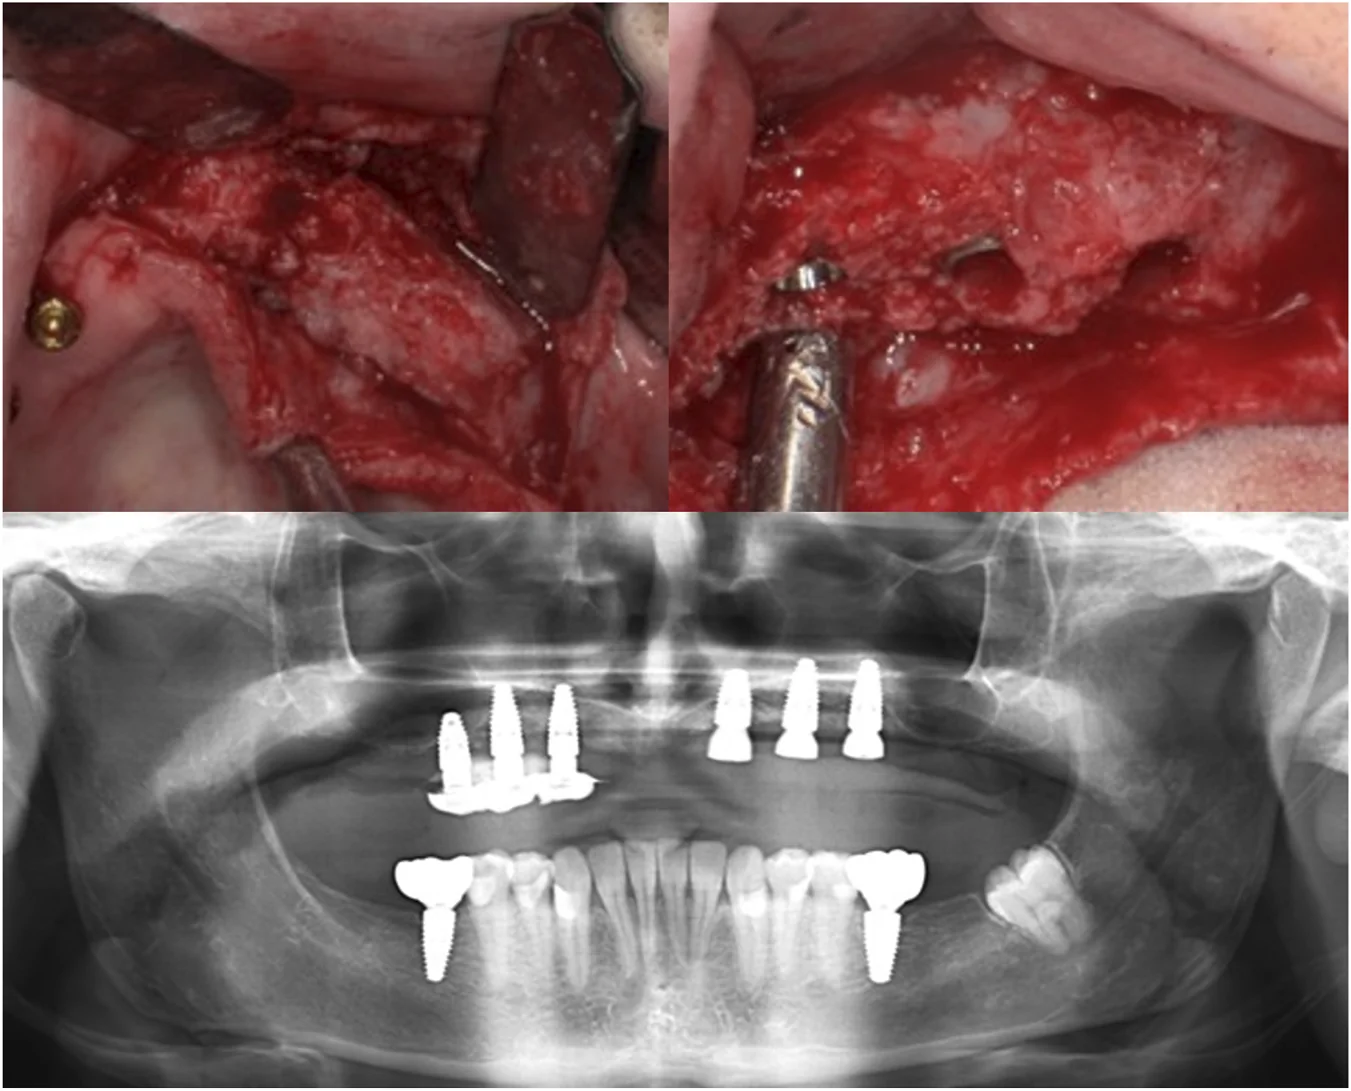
Case 2: implant placement and 6 months post-implant placement. Clinical photographs (top right and left) showing the quality of the bone at the time of dental implant placement. Panoramic radiograph (bottom) showing osteointegration of the three dental implants 6 months post placement.
